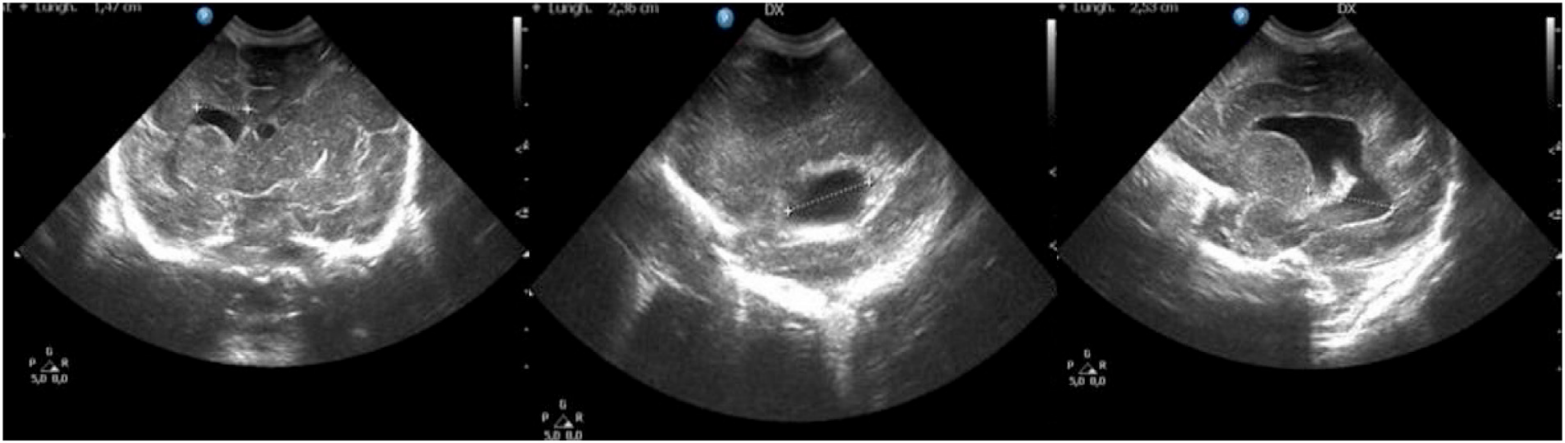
Cerebral Ultrasound performed after birth. Cerebral ultrasound performed on the first day of life both in coronal and sagittal images. It shows left cerebral hemisphere prevailing on the right, a poroencephalic area of 2.3 cm of diameter in the right side, with “ex vacuo” ventricle enlargement (Ventricular Index1.4 cm. Occipital Thalamus Distance 2.5 cm) till Silvian fissure.
